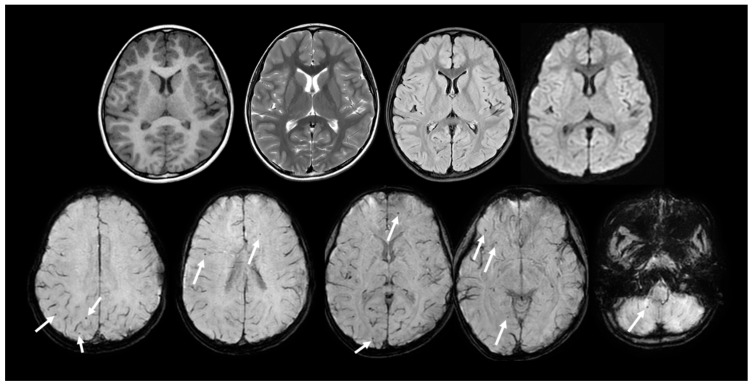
Representative structural findings on sedation-free ultrafast brain MRI in Fontan patients. Upper row: Representative sedation-free ultrafast brain MRI sequences (T1-weighted image, T2-weighted image, FLAIR and DWI) from a Fontan patient without significant structural abnormalities. Lower row: Susceptibility-weighted imaging from the ultrafast protocol demonstrating multiple small hypointense foci (arrows), consistent with cerebral and cerebellar microbleeds. These findings exemplify subclinical microvascular injury that may contribute to cognitive vulnerability in this population. MRI, magnetic resonance imaging; FLAIR, fluid-attenuated inversion recovery; DWI, diffusion-weighted image.
